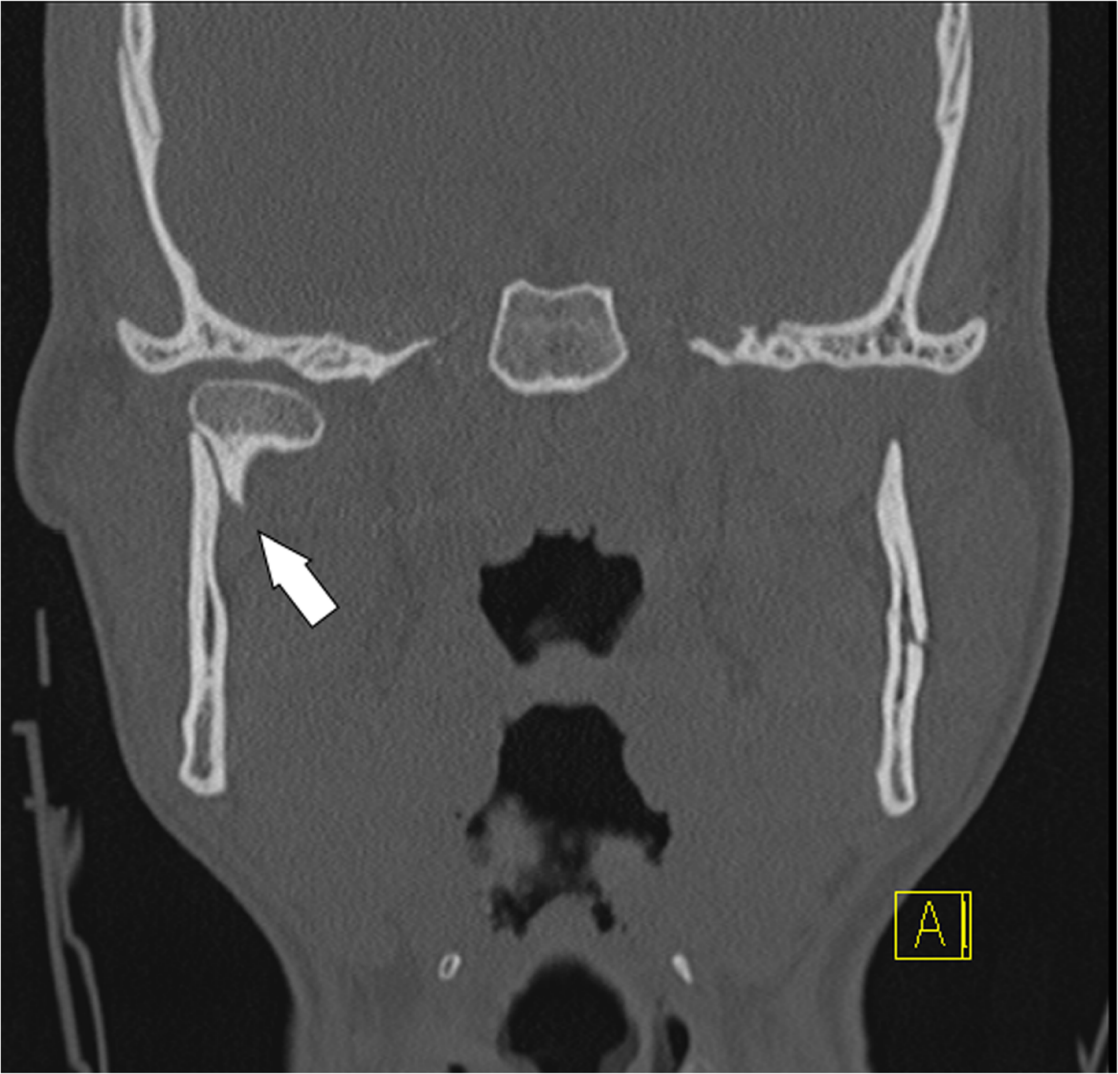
Condylar process fracture. Deviation. Computed tomography coronal section shows that the condylar head remains within the glenoid fossa and the contact between the bone fragments is not completely lost (arrow)
